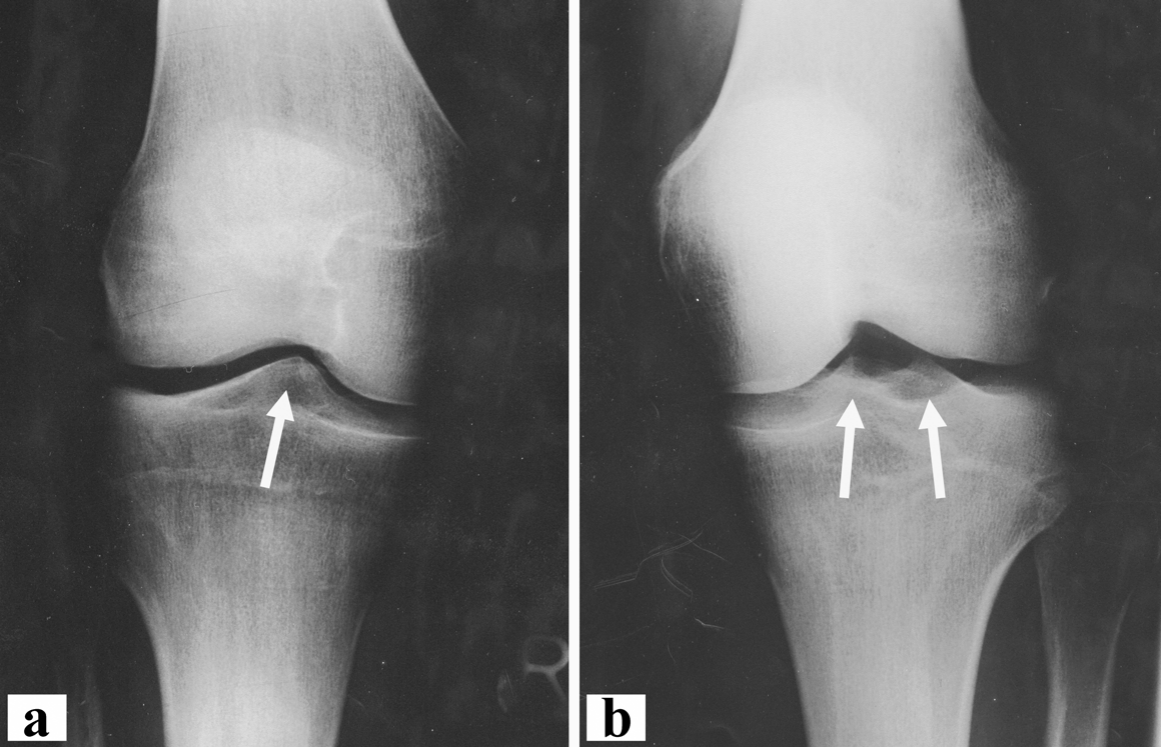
Radiographs of both knee joints. Anteroposterior radiographs of the affected (a) and contralateral (b) knee joint. The "dromedar-sign" with only one tibial spine is visible (a - arrow) as opposed to the normal radiological finding with two spines (b - arrows). If a "dromedar-sign" is visible on plain radiographs the arthroscopic surgeon should be alert of an aplasia of the cruciate ligaments.
